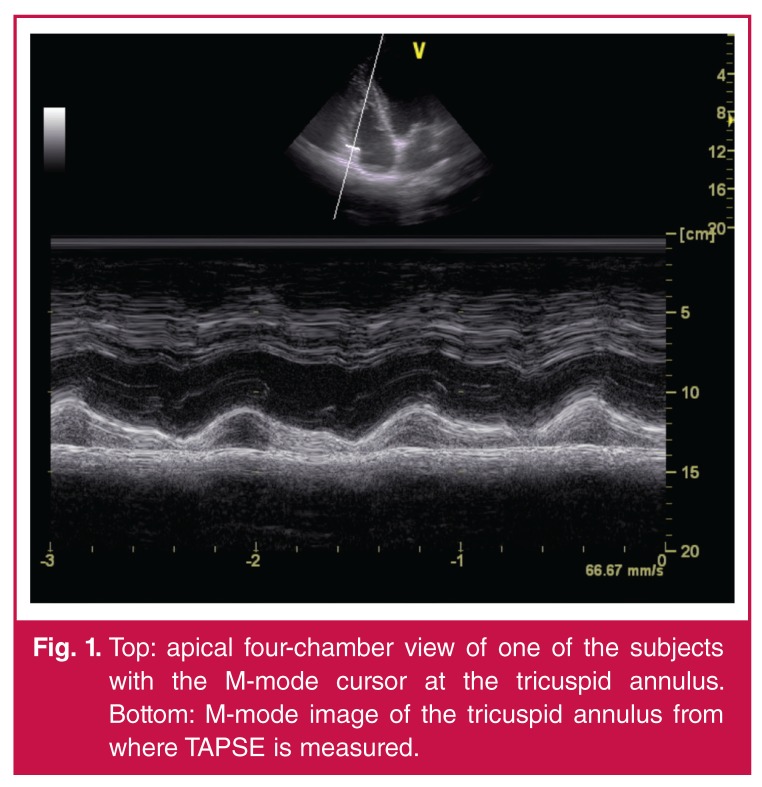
Top: apical four-chamber view of one of the subjects with the M-mode cursor at the tricuspid annulus. Bottom: M-mode image of the tricuspid annulus from where TAPSE is measured.
